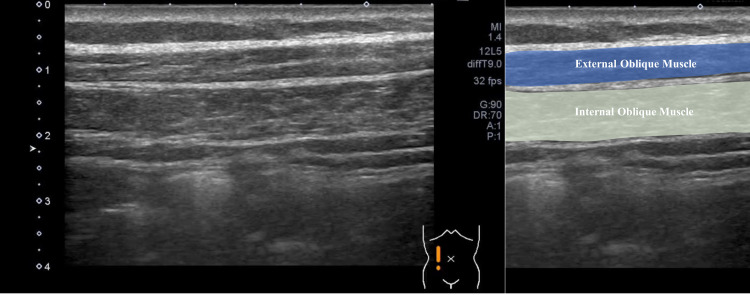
Muscle ultrasonography. Long-axis image of the oblique muscles.
